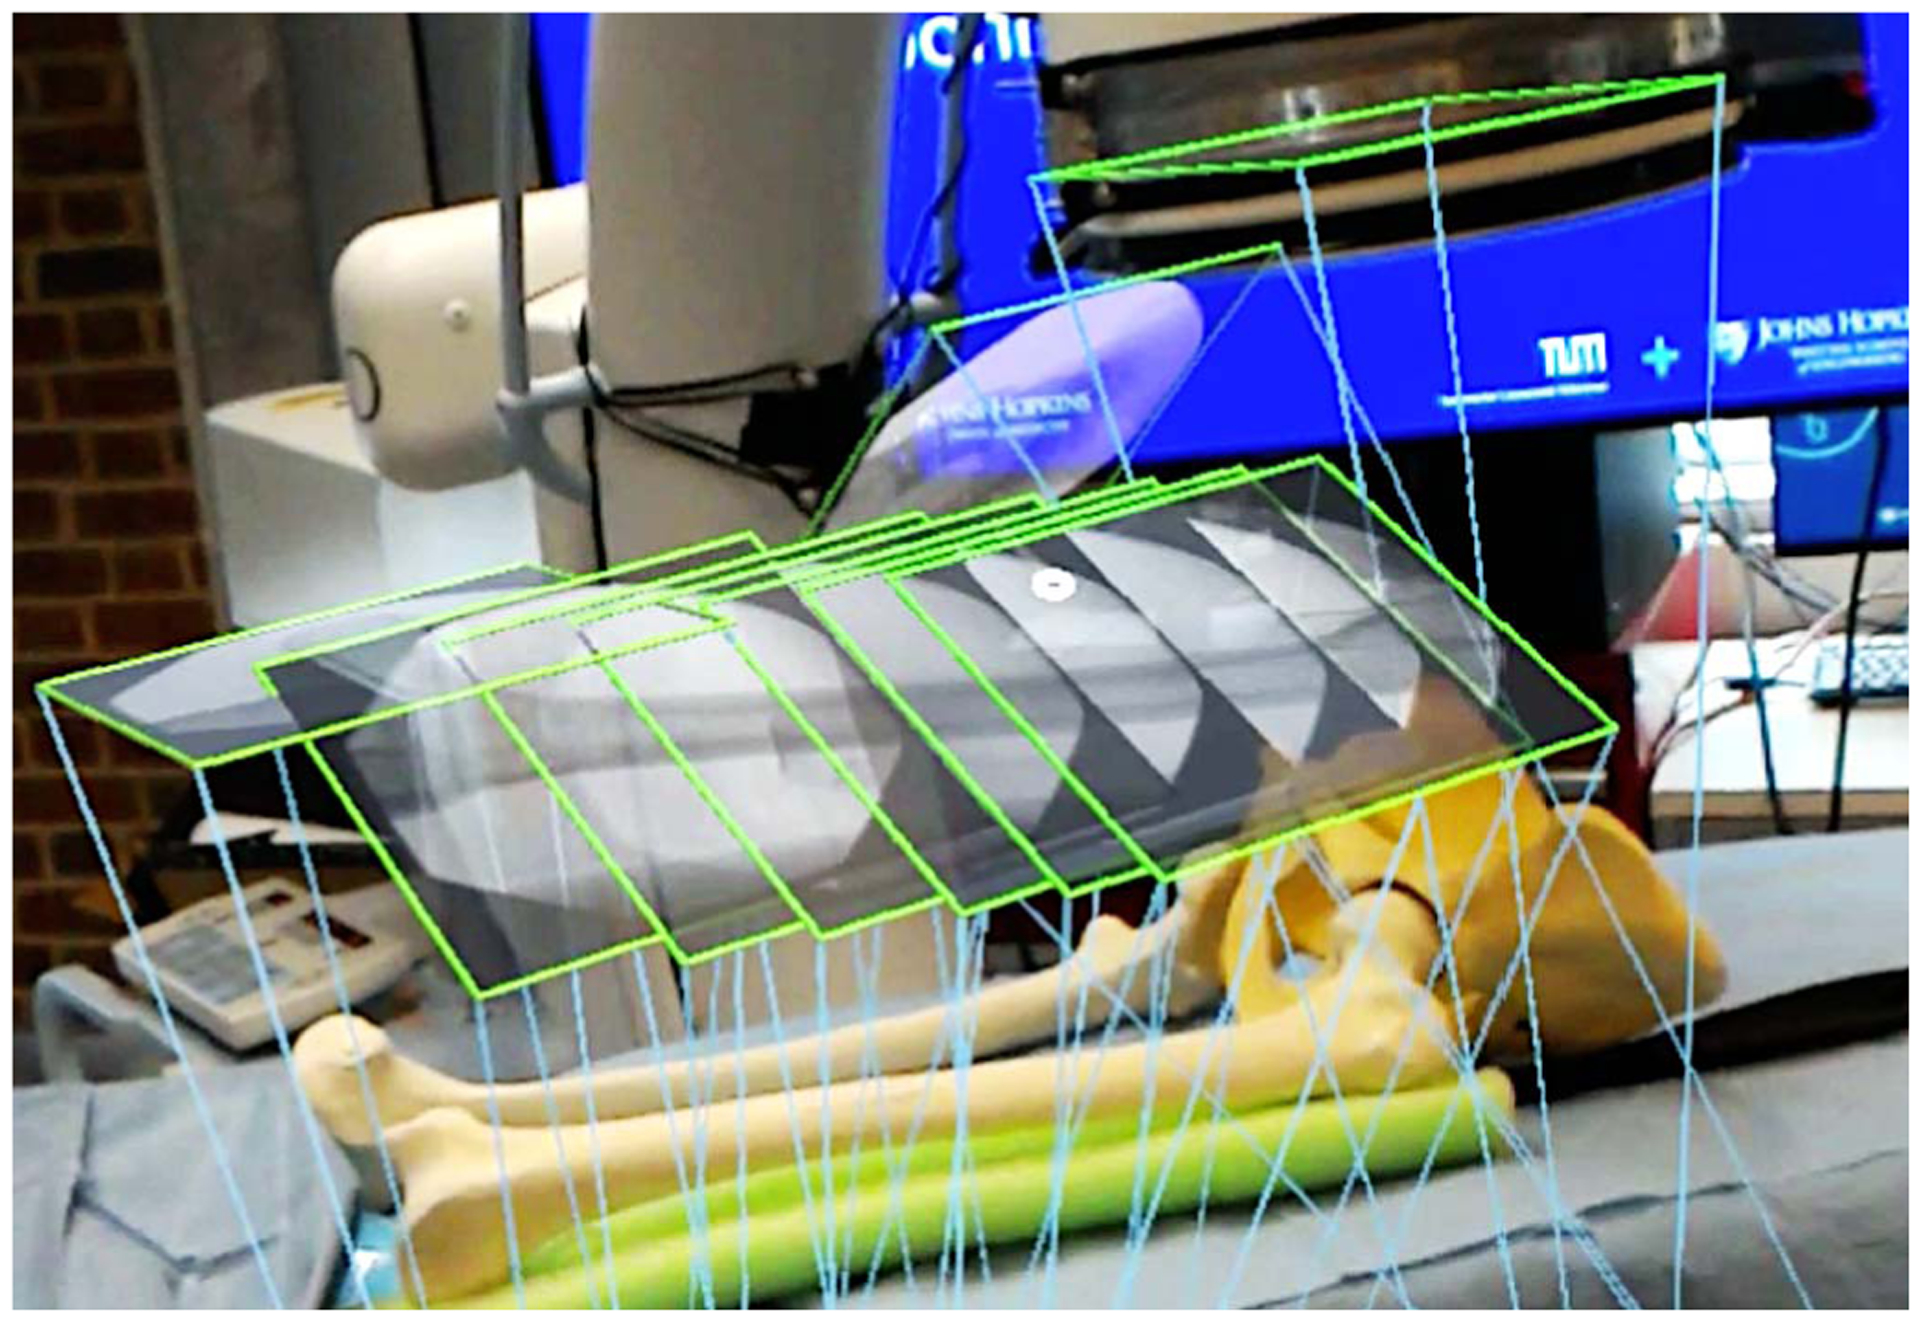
The interlocking of multiple X-ray frustums enables visualization of large anatomical structures. In this figure, multiple images are acquired on a co-linear trajectory and are locked to each other to form a quasi-panoramic view of the bone.
